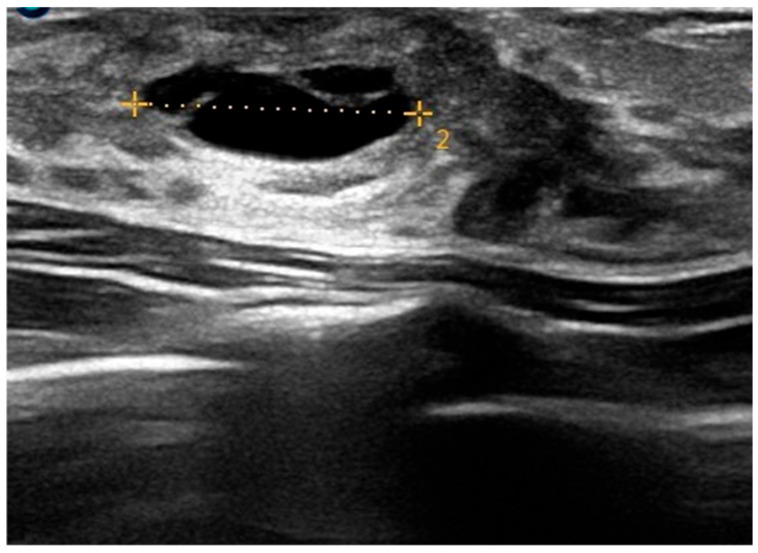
Ultrasound examination at 12-month follow-up confirming the presence of an anechoic lesion with regular margins, measuring approximately 15 mm in maximum diameter, stable over time.
